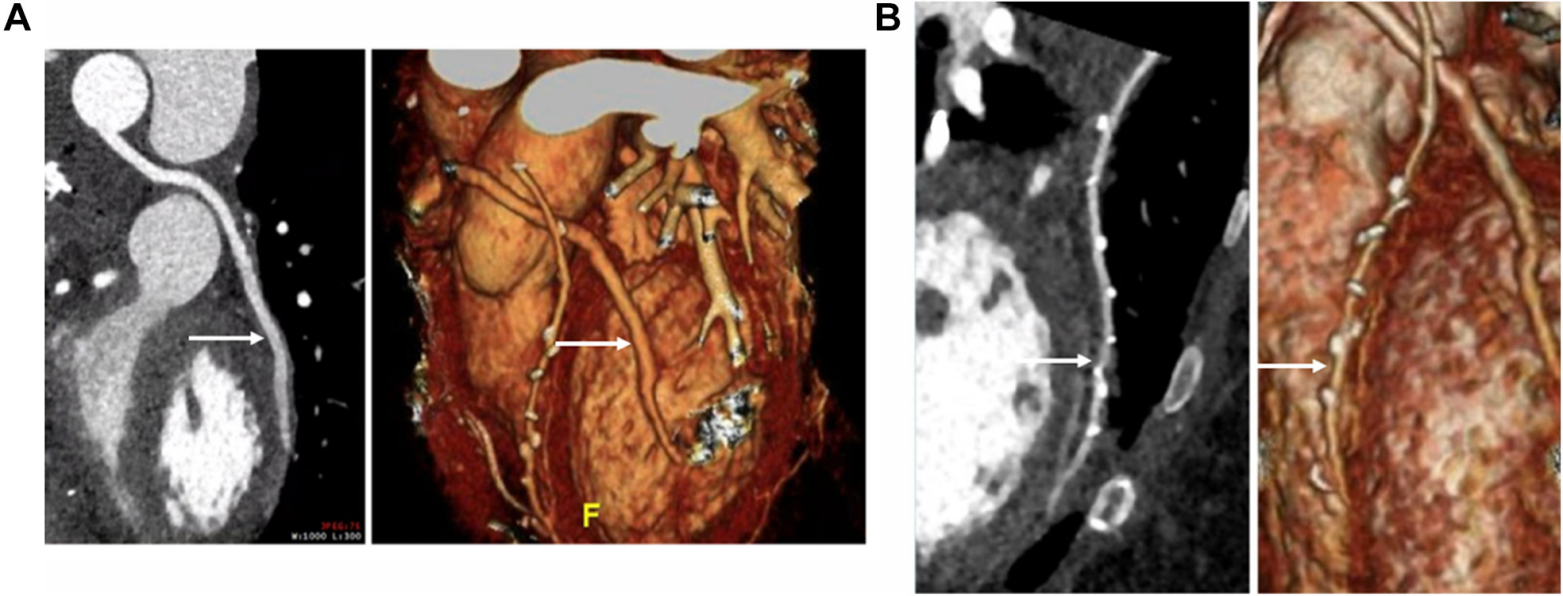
Postoperative Imaging (A) Three-dimensional computed tomography reconstruction of the patent saphenous vein to ramus intermedius graft (indicated by white arrows) with long distal anastomosis. (B) Three-dimensional computed tomography reconstruction showing the patent left internal mammary artery to distal left anterior descending graft (indicated by white arrows).
